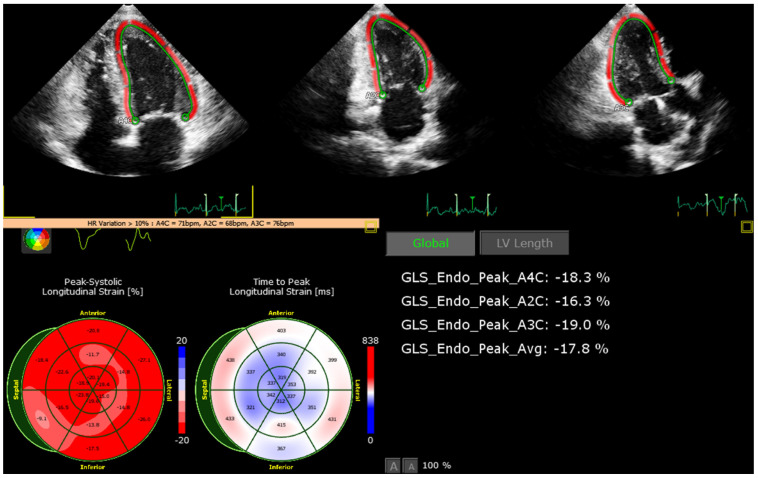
Strain imaging showing an average GLS at the lower limit of normal. Strain imaging was performed revealing that this patient had an average global longitudinal myocardial strain (GLS) at the lower limit of normal (−17.8%). This finding highlights that strain imaging may be a useful tool to investigate myocardial bridging, as it showed diagnostic information otherwise not available by conventional echocardiography. GLS: global longitudinal strain.
